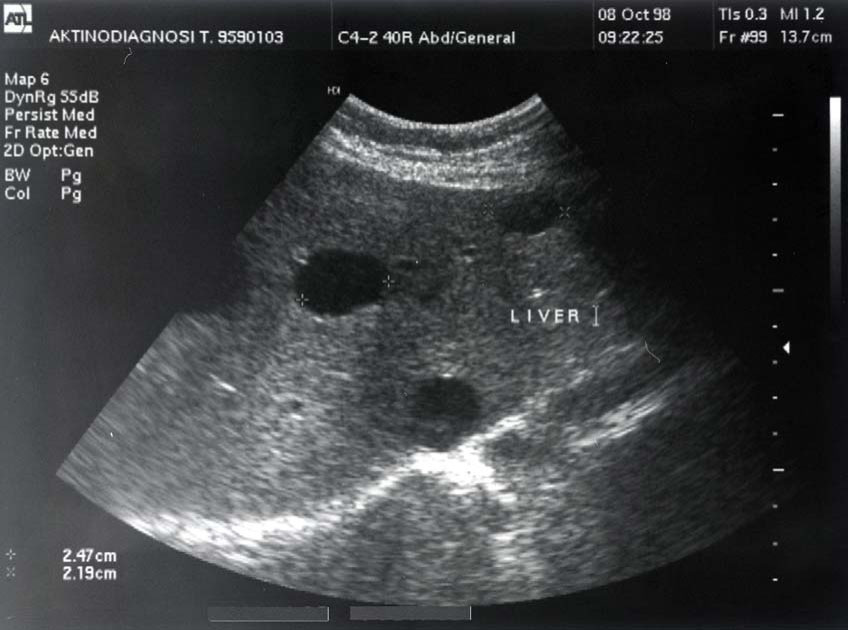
Appearance via ultrasonography of simple liver cysts two years before the patient's latest admission.
